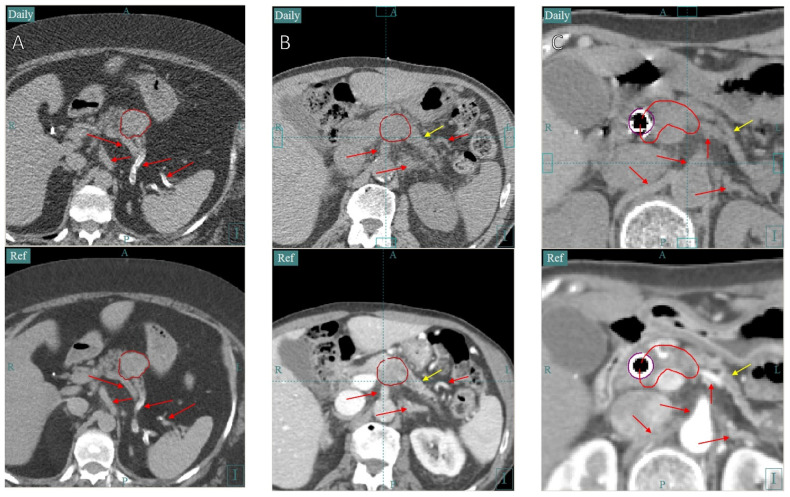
This figure demonstrates the ability to visualize with CTOR images the pancreas and the surrounding anatomy necessary to align with the GTV (red contour). The top row is the CTOR images and the bottom is the planning CT images. Comparing nearby blood vessels (red arrows) and the pancreas shape (yellow arrows) between the two images can be used to achieve good alignment in the superior–inferior direction, which is often the hardest, and can aide in the alignment in other directions as well. Calcifications within blood vessels (as seen in (A,B)) can also be a useful tool. Stents (purple contour in (C)) can be used to assist with alignment, but should be used with caution, as they may move relative to the tumor. The window/level for all the images is 400/800 HU.
